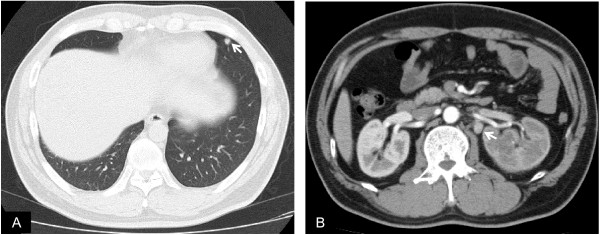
Preoperative CT images showed a nodule in the left lung (A, arrow), and para-aortic lymph node enlargement (B, arrow).
